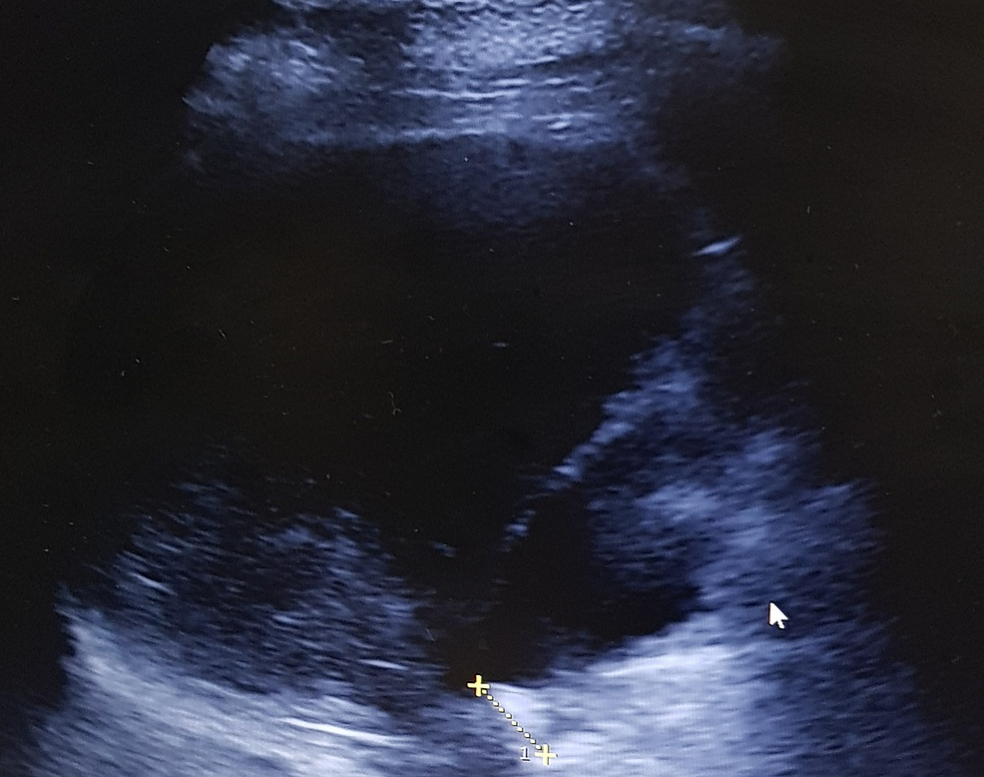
Abdominal ultrasound showing the thickened gallbladder containing echogenic material (arrow) with stone impacted at its neck.
